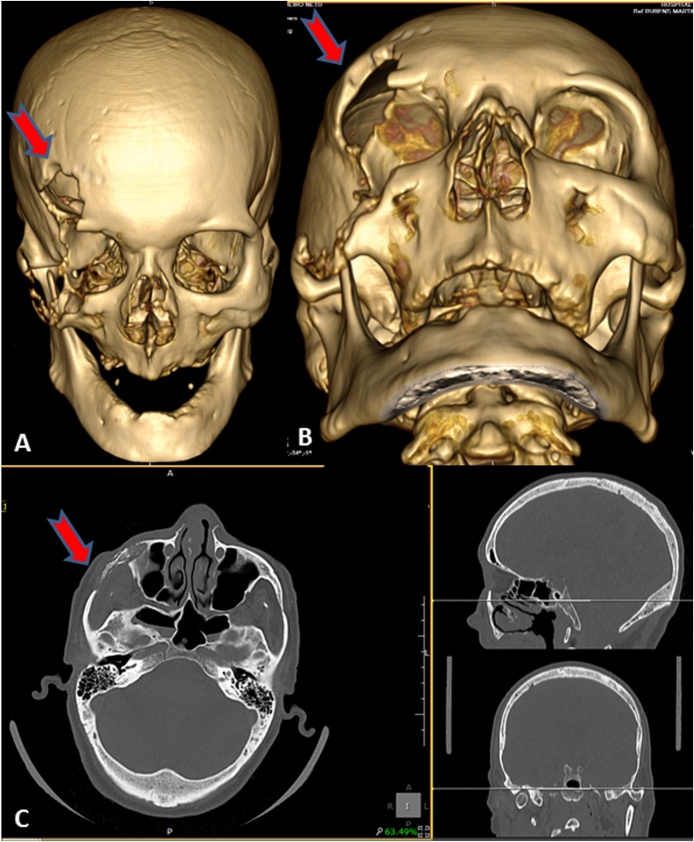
A and B - Computed tomography in three-dimensional reconstruction showing lesion in the right frontotemporal region (red arrows). C - Axial section of tomographic examination indicating destruction of the right orbital cavity (red arrow).
